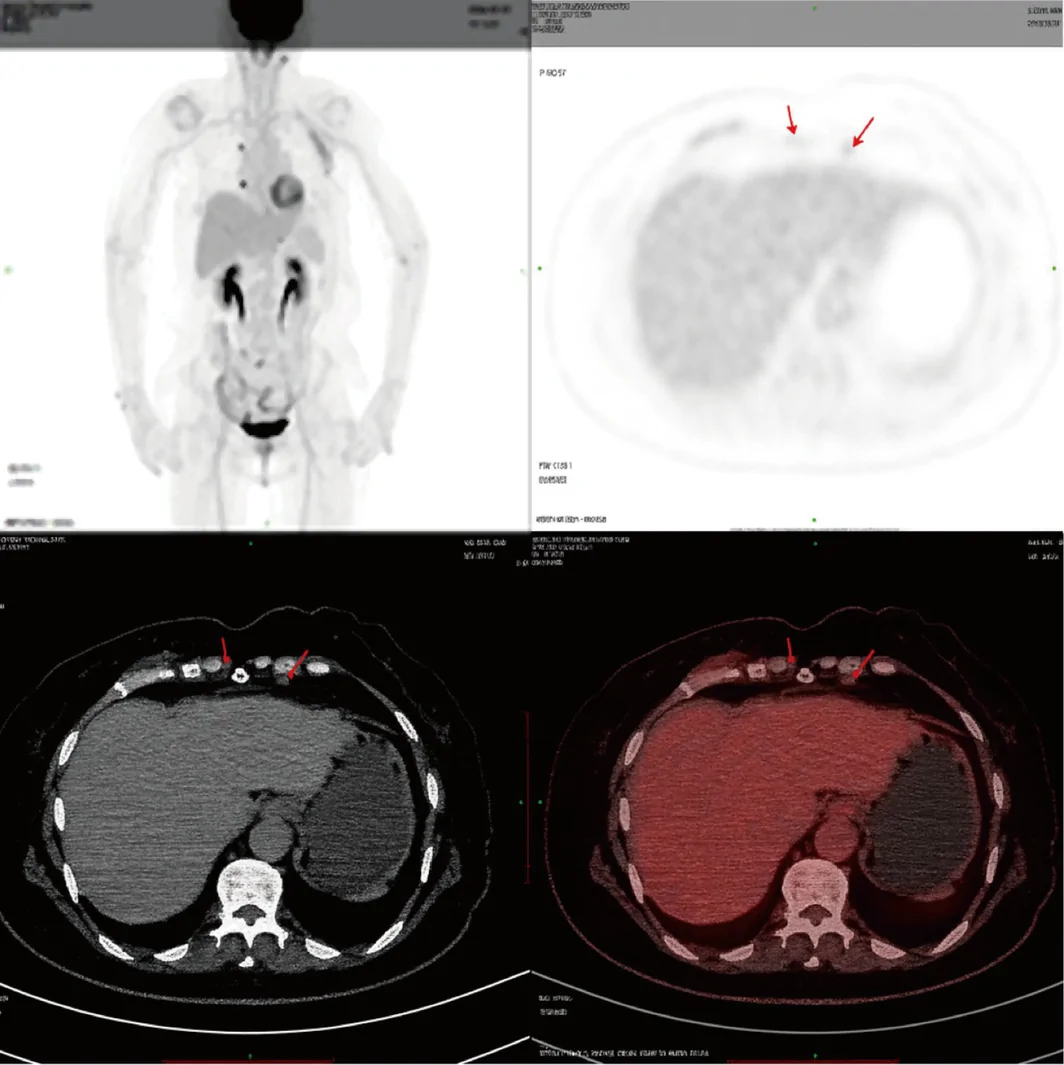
PET‐CT demonstrating disseminated nodal metastases of ovarian tumor.
